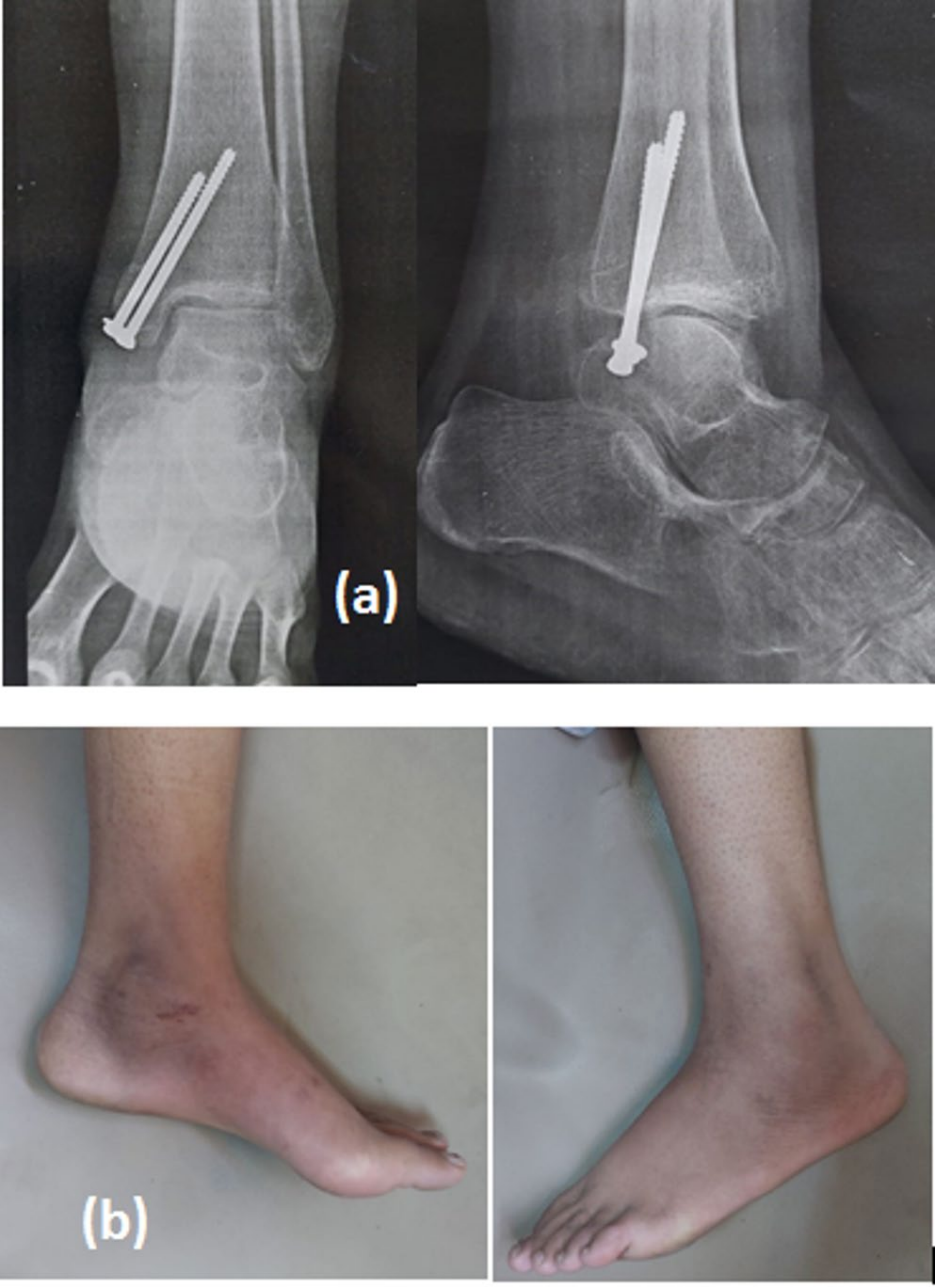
Follow-up: a radiographs showing complete union at final follow up. b Skin condition at final follow up showing minimal scar medially with no scars laterally
